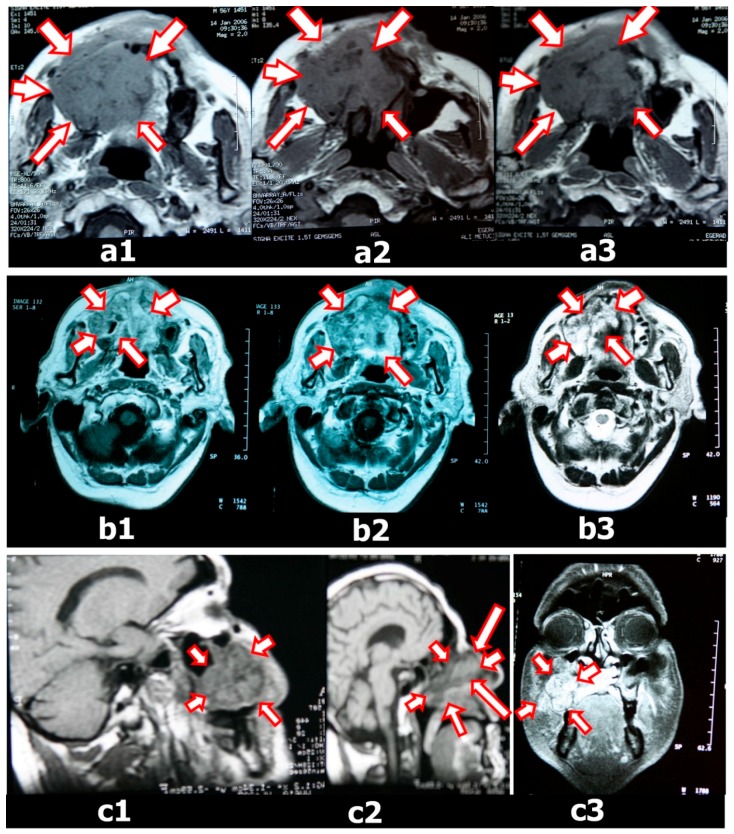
Axial and Coronal MRI weighted images showing a lesion of the maxillofacial region. (a1–3) MRI images of a palatomaxillar tumor on the horizontal plane before chemotherapy and radiotherapy (white arrows with red border); (b1–3) MRI images of the palatomaxillar tumor on the horizontal plane after chemotherapy and radiotherapy (white arrows with red border); (c) MRI images of the palatomaxillar tumor on the sagittal (c1,2) and frontal (c3) plane after chemotherapy and radiotherapy (white arrows with red border).
